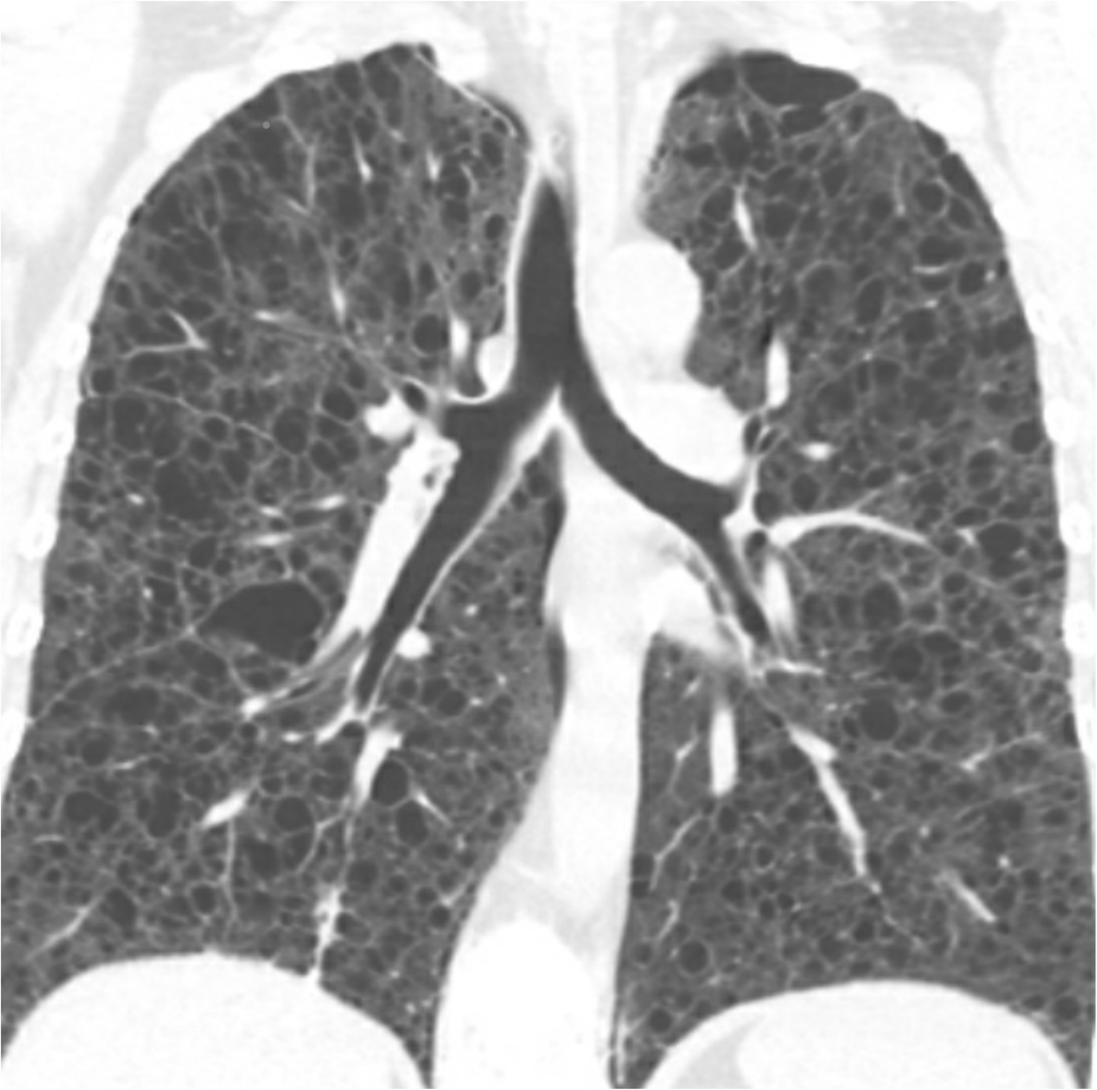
Coronal CT reformatted image of the chest shows diffuse severe pulmonary involvement by thin-walled cysts. Although several cysts are small (2–5 mm), the majority are much larger, measuring up to 12 mm. Note variable cyst shapes including polygonal shapes and a diffuse involvement of the lungs
